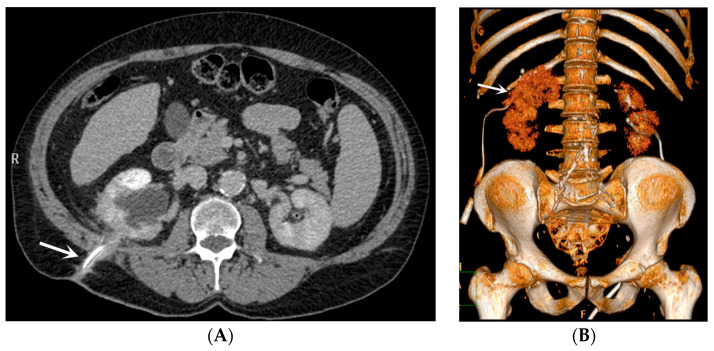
Dislodged right nephrostomy catheter. Axial CT scan (A) and volume-rendering CT image (B). The device (arrow) is seen in the loin soft tissues. The renal enhancement is heterogeneous and the hydronephrosis persists.
